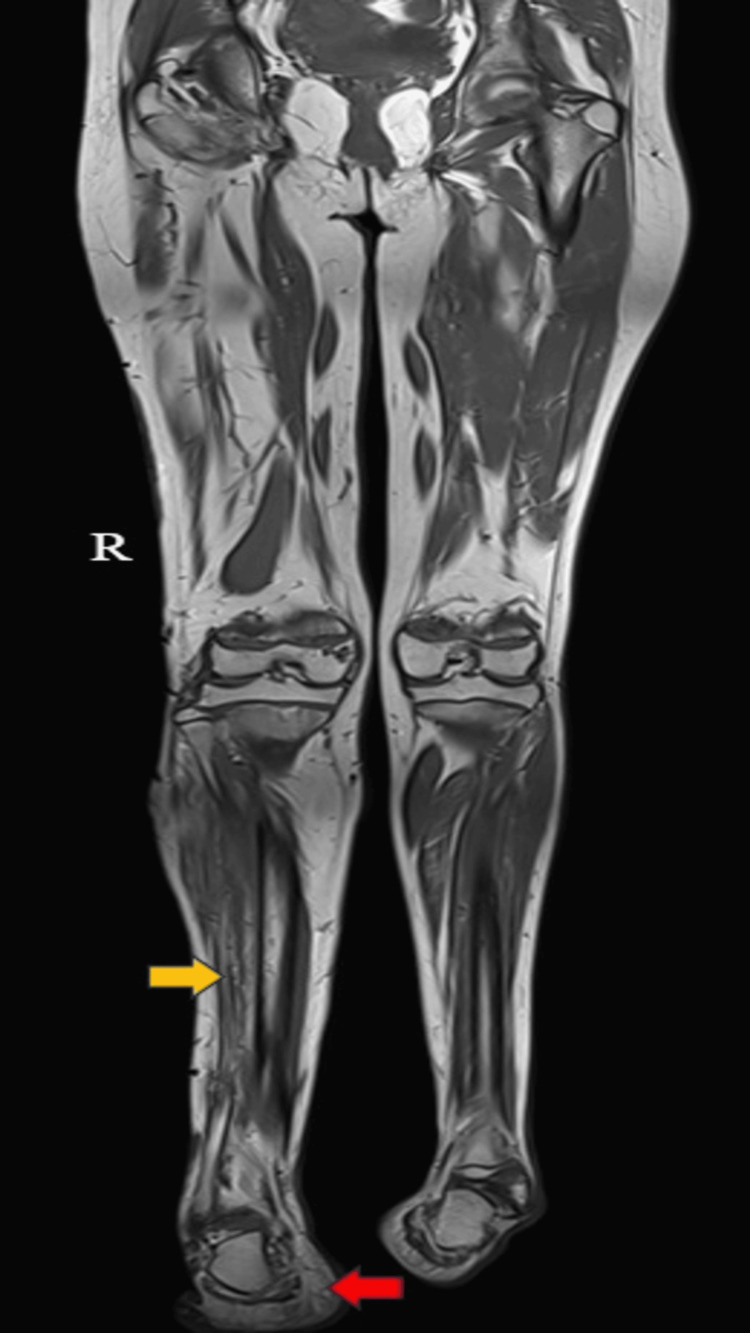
An MRI of the bilateral lower limb axial T1WI shows an enlarged and elongated right lower limb (yellow arrow) and inversion of bilateral feet (red arrow). T1WI: T1-weighted imaging
